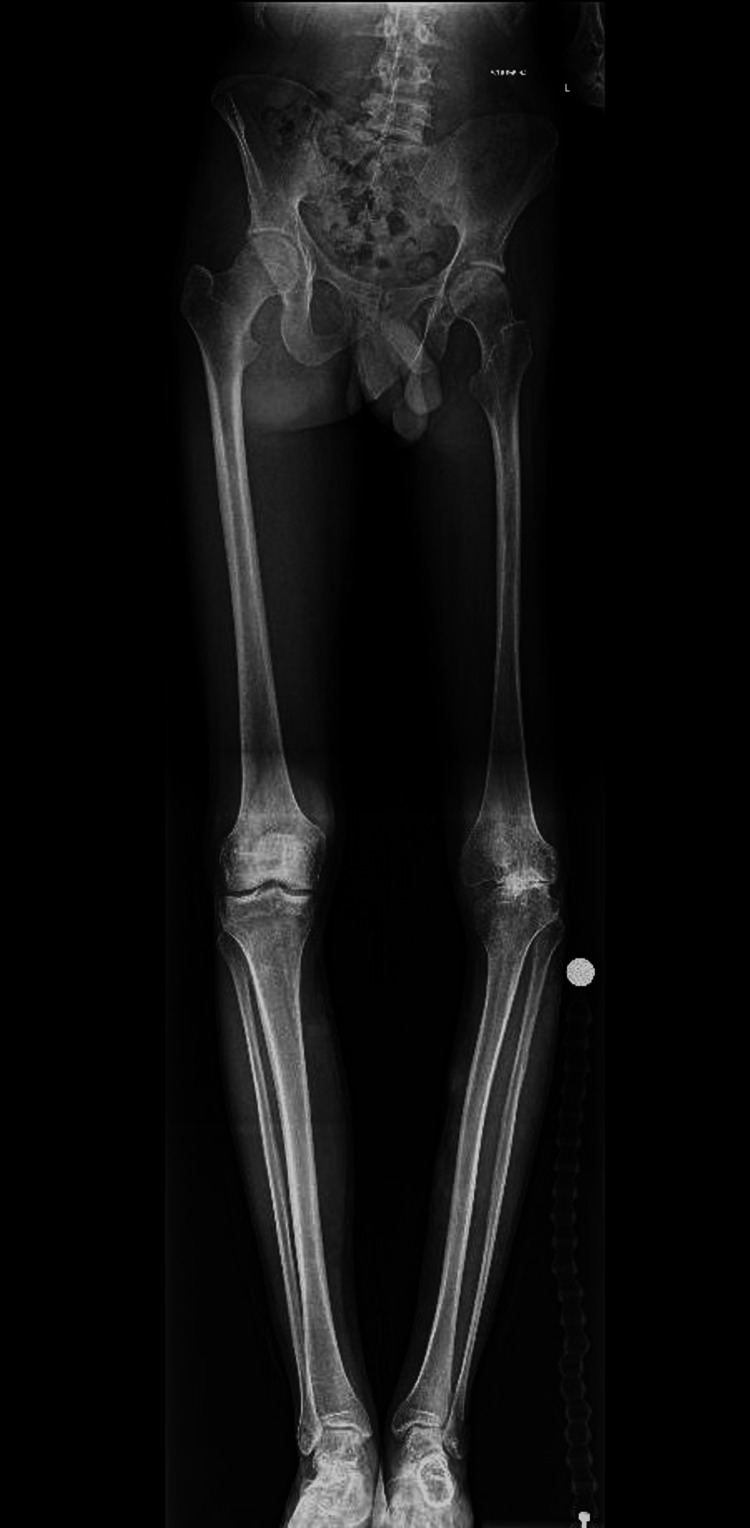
Long-leg X-ray demonstrating shortening of the left lower limb, varus deformity, and resulting hip tilt.
